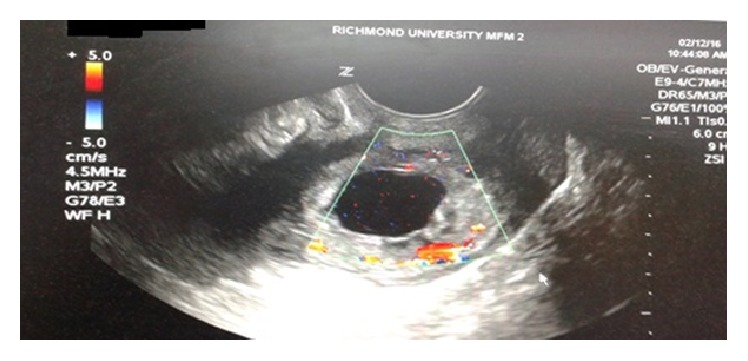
Vascularization around the gestational sac demonstrated by Doppler color flow.
